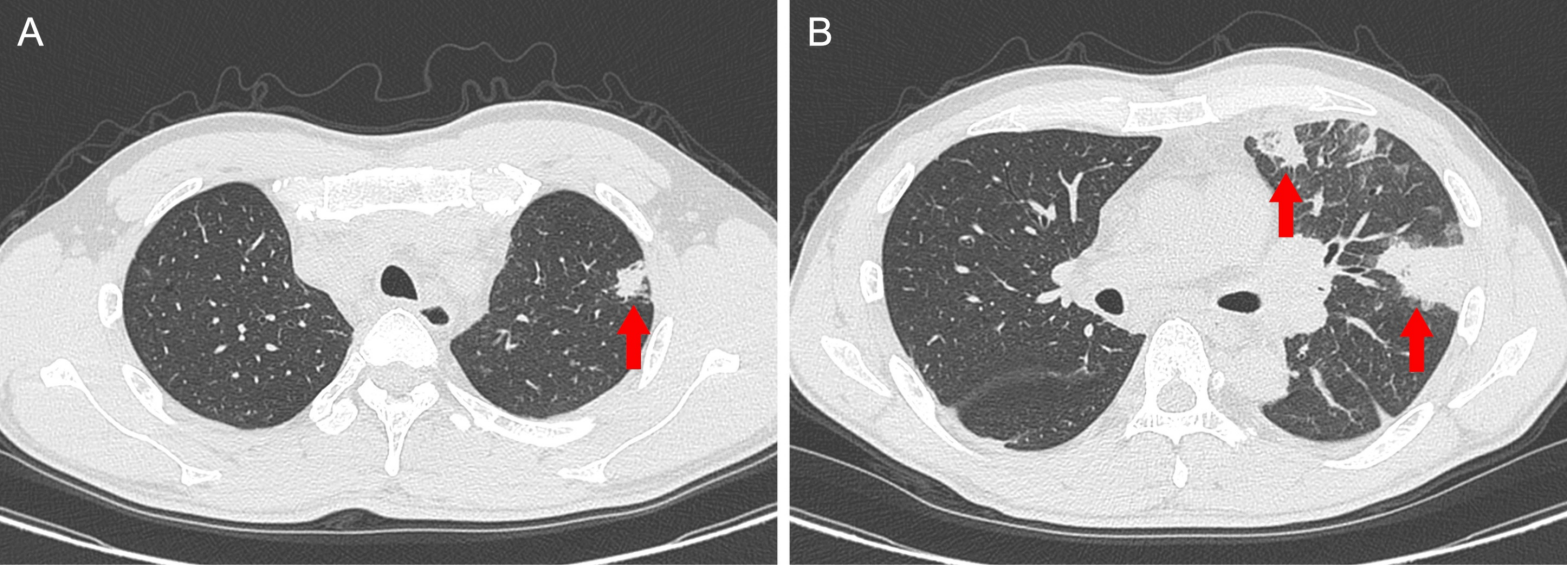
CT findings Chest CT shows an irregularly shaped nodule in the left upper lobe (A, arrow), accompanied by multiple wedge-shaped opacities in the left lung parenchyma (B, arrows).
